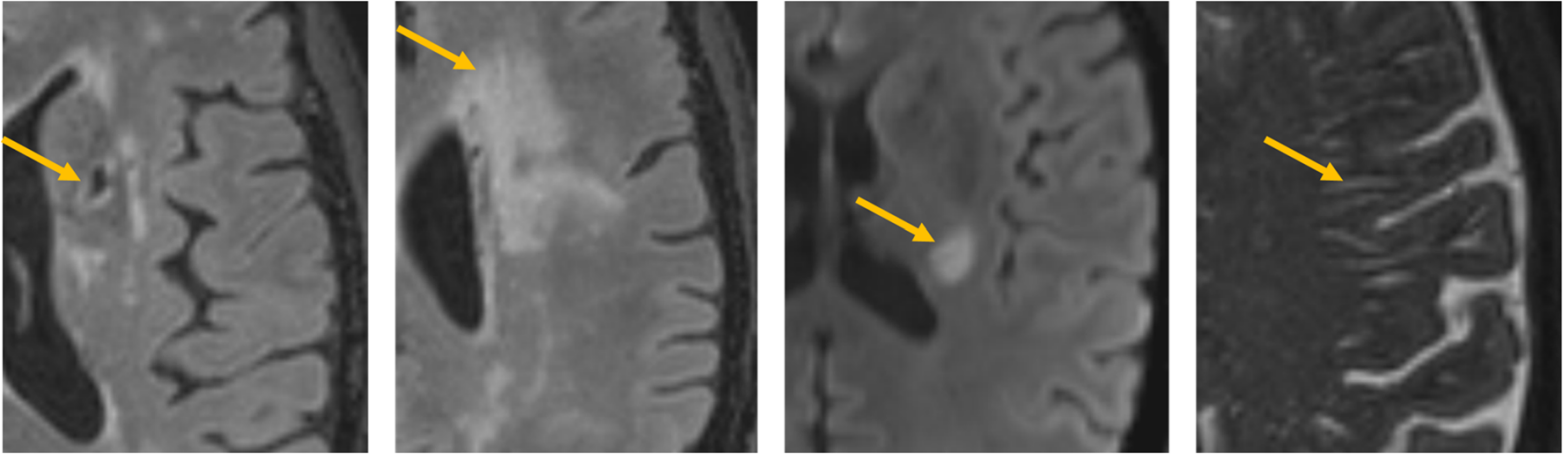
Examples of the appearance of SVD features in human, from left to right: recent small subcortical (i.e. acute lacunar) infarct (DWI), WMH (FLAIR), lacune (FLAIR) and enlarged perivascular spaces (T2-w) indicated by yellow arrows
